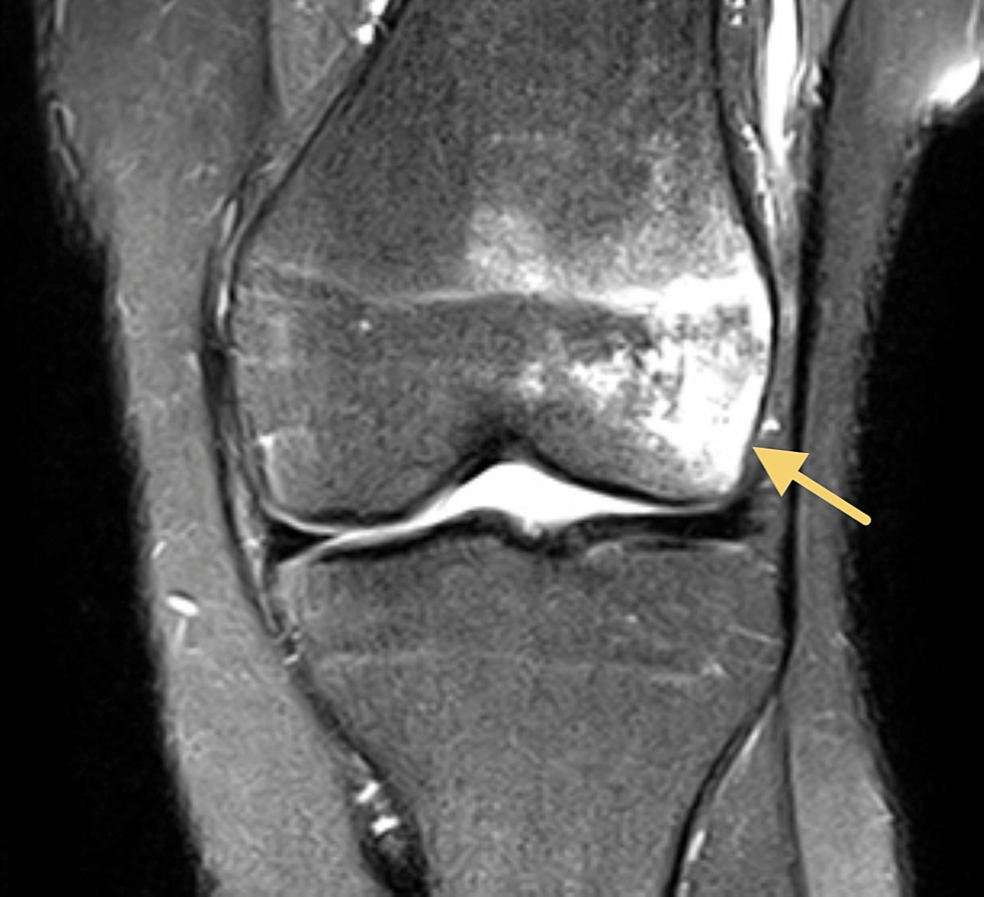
MRI for the first patient, revealing a bony contusion of the lateral femoral condyle The arrow indicates the bony contusion of the lateral femoral condyle.
